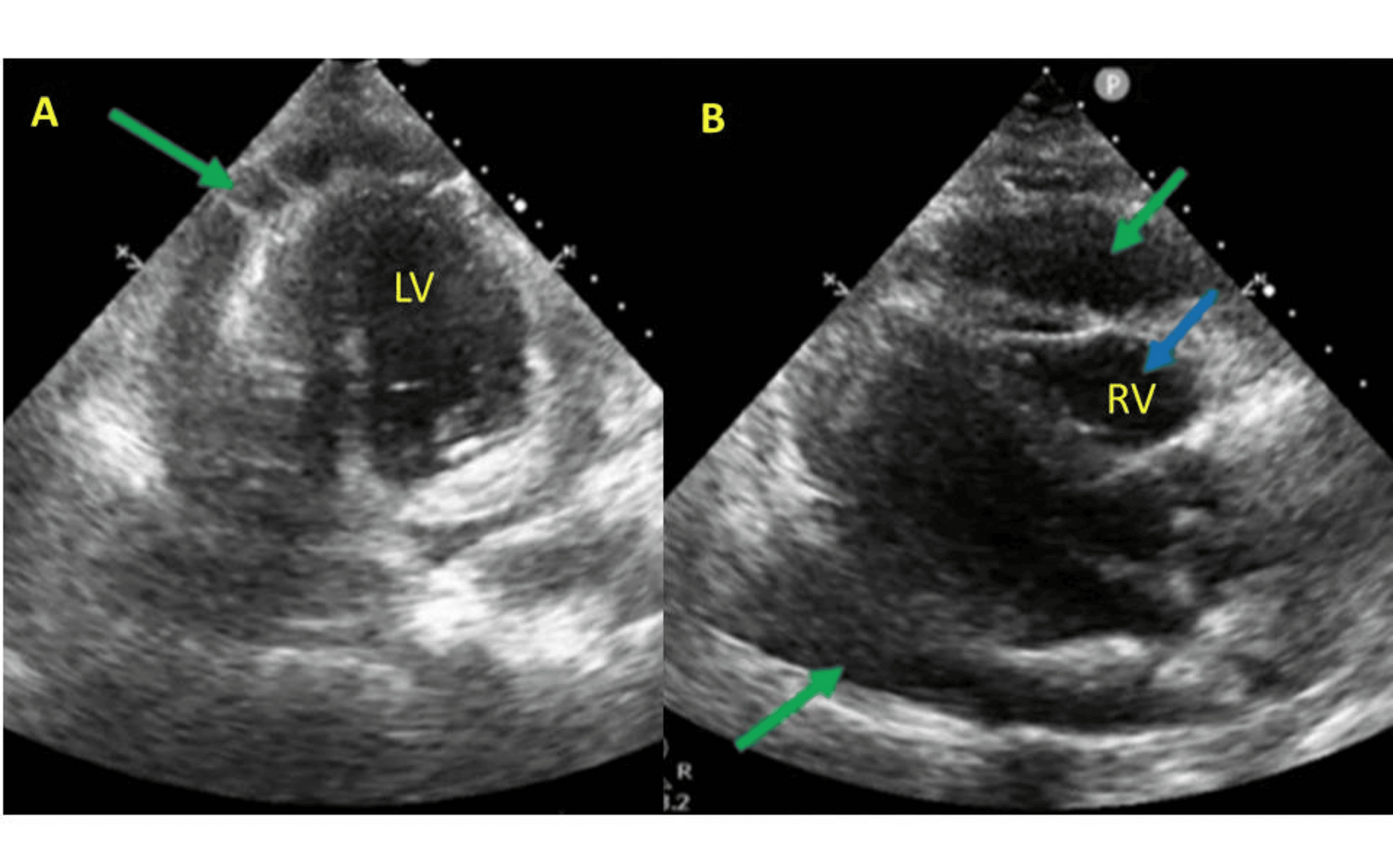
Point-of-care echocardiographic findings of cardiac tamponade at presentation A: Apical four-chamber point-of-care echocardiographic view obtained at Emergency Department admission, demonstrating a large pericardial effusion (green arrow). B: Parasternal long-axis point-of-care echocardiographic view showing a large pericardial effusion (green arrows) with echocardiographic features of cardiac tamponade, including diastolic right ventricular collapse (blue arrow). These images were acquired during hemodynamic instability; formal Doppler and M-mode analysis were not performed at this stage. LV, left ventricle; RV, right ventricle
